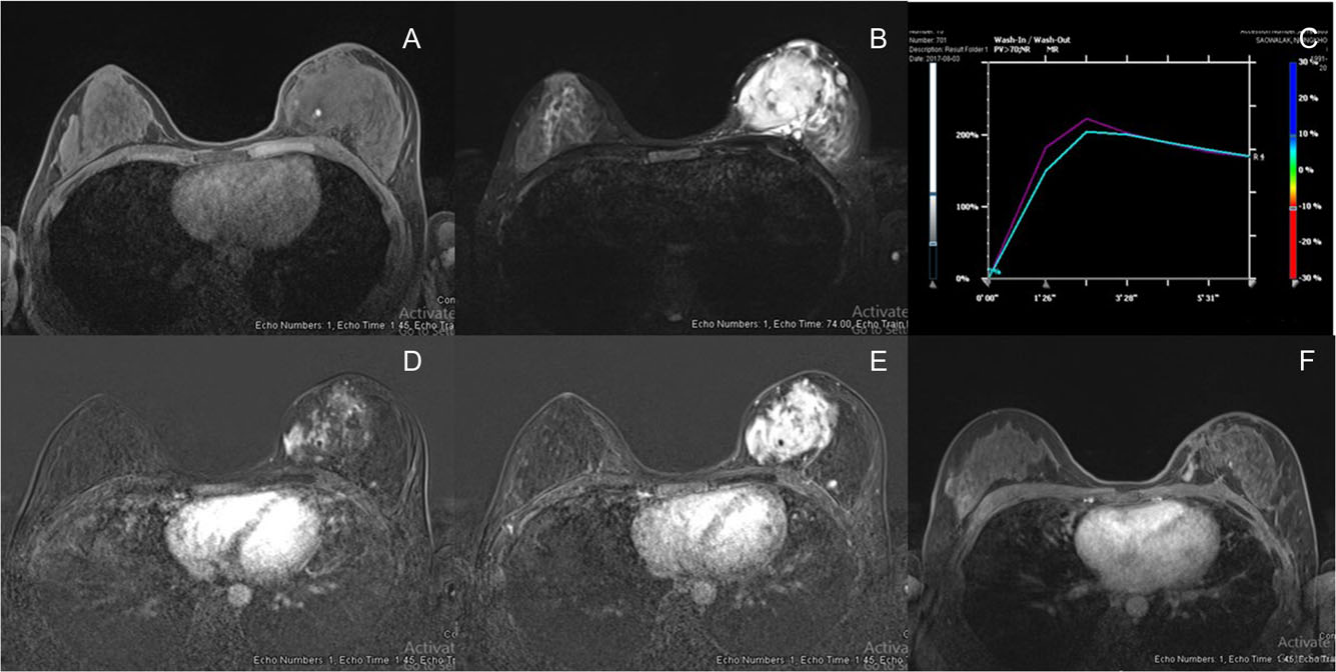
(A) Breast MRI showed isosignal intensity in T1WI with hemorrhagic spot (arrow). (B) Breast MRI showed strongly high signal intensity in T2WI. (C) Signal time curve showed type 2 and type 3 kinetic curve pattern but type 2 in majority. (D) Breast MRI showed rapid arterial enhancement in early arterial phase. (E) Breast MRI showed mixed plateau and washout enhancement in delayed phase. (F) Breast MRI post radiation showed interval decreased in size, currently showed slow arterial enhancement with persistent enhancement on delayed phases (type I kinetic).
